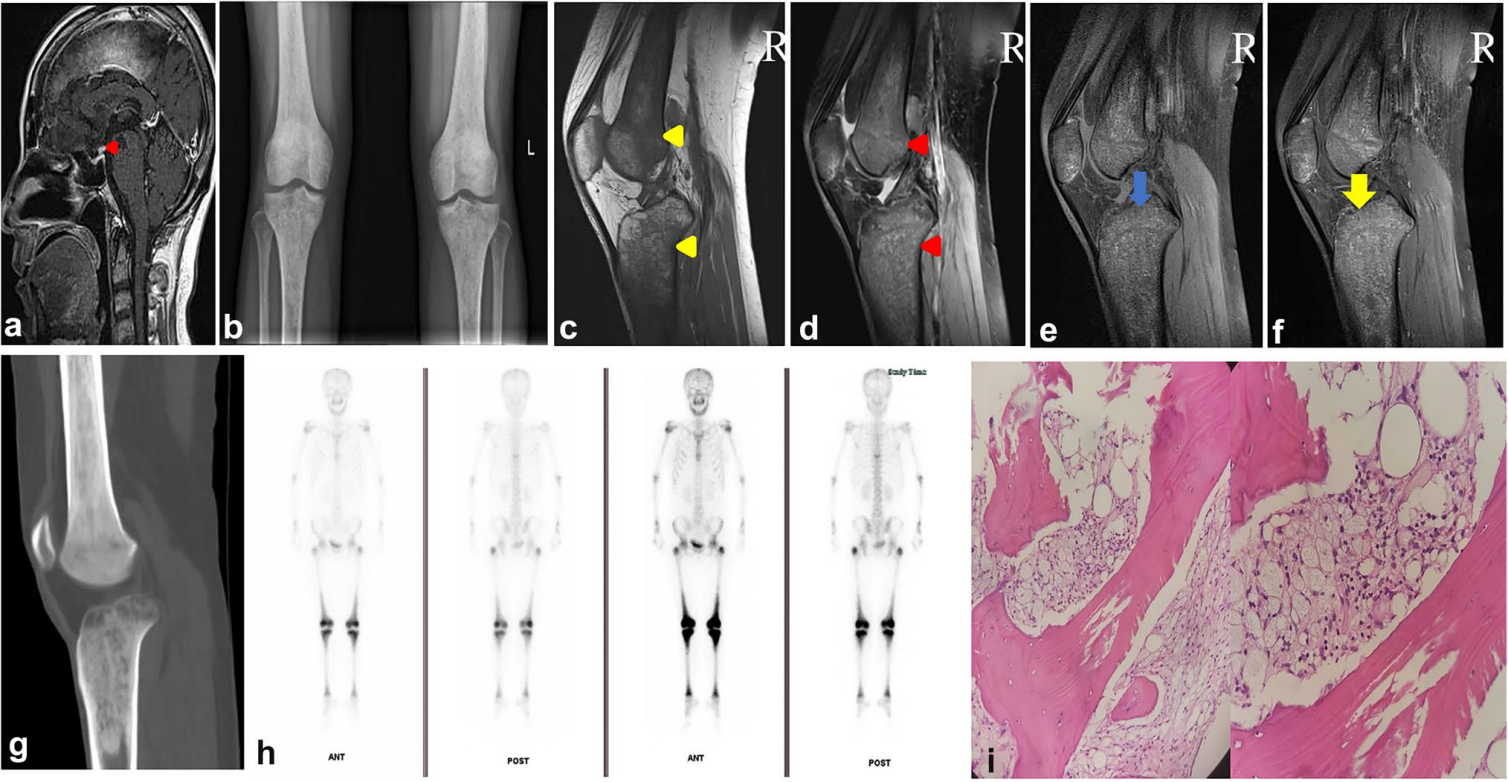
Magnetic resonance imaging (MRI), computed tomography (CT), and X-ray images. a MRI of enlarged pituitary stalk (red triangle). b Anteroposterior radiographs of bilateral knee joints showing extensive bone destruction of bilateral upper tibia and lower femur. c MRI showing long T1 signal of lower femur and upper tibia (yellow triangle). d MRI of lower femur and upper tibia showing long T2 signal of fat compression (red triangle). e MRI showing fat suppression (blue arrow). f MRI enhancement scan showing obvious enhancement (yellow arrow). g CT of right knee showing high-density image in bone marrow cavity. h
